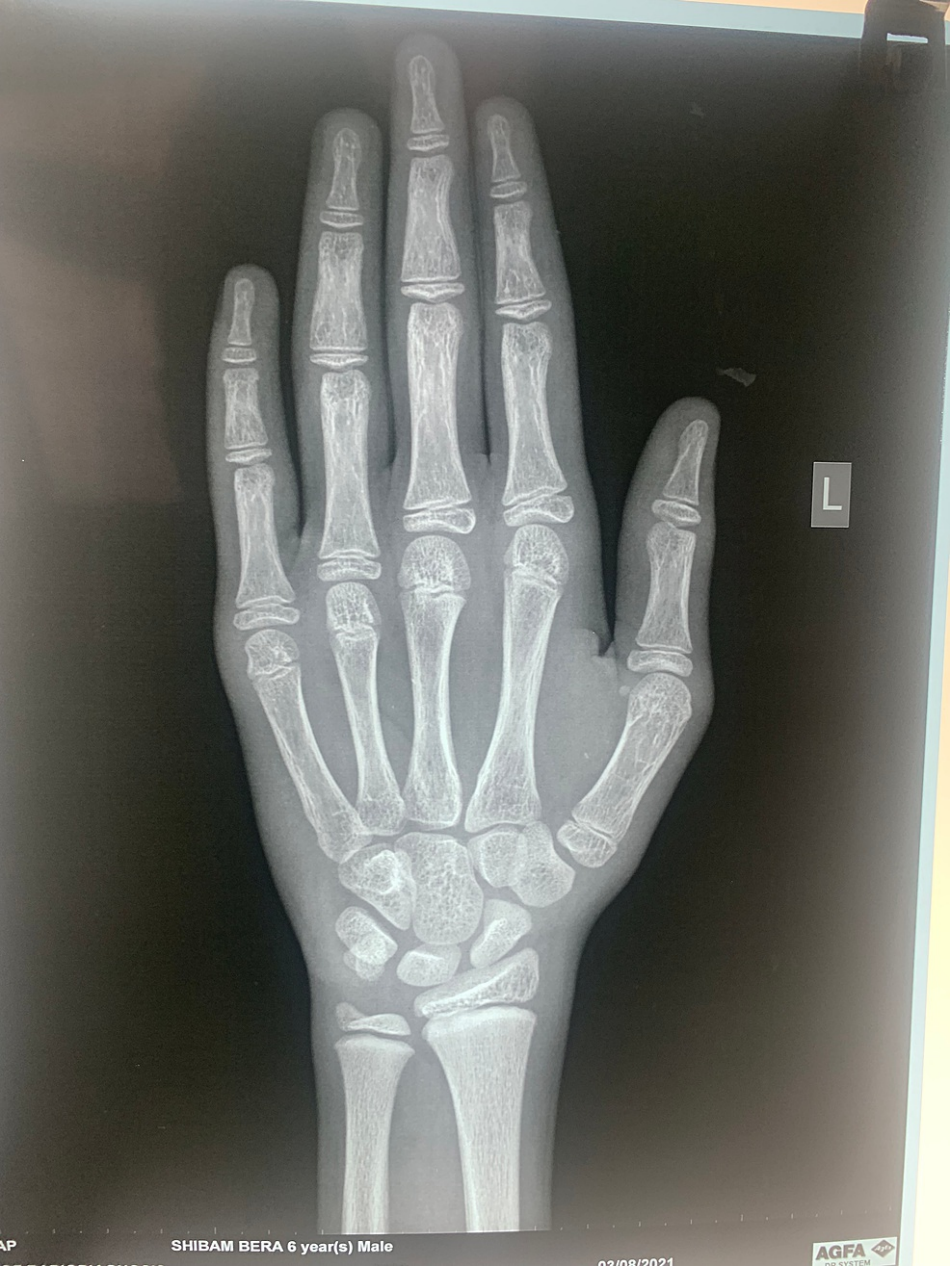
X-ray of left hand showing bone age of 12 years by Greulich and Pyle chart.
